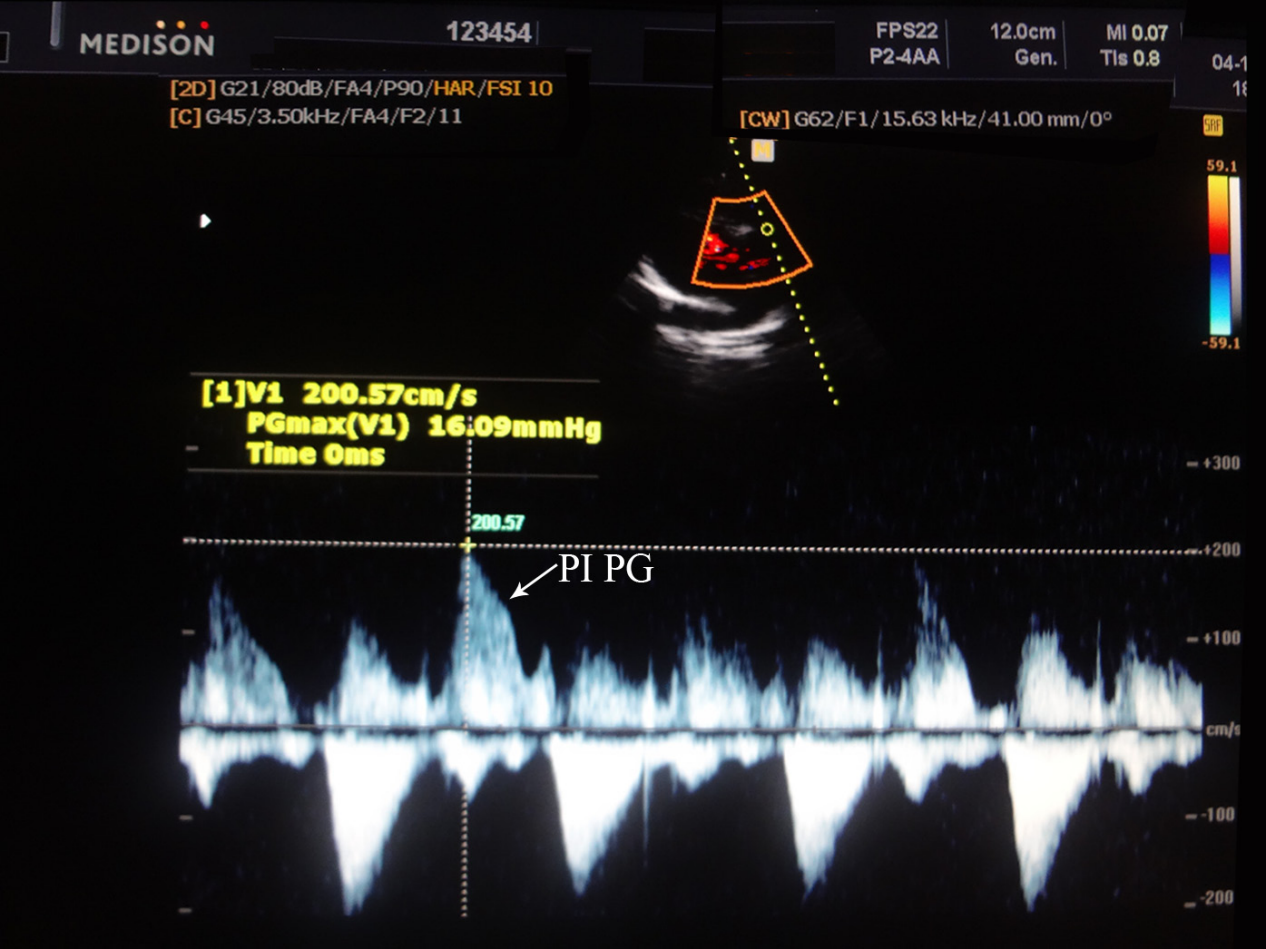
Continuous wave Doppler tracing of the pulmonary valve of the patient by transthoracic echocardiography at 2 years’ follow up shows mild pulmonary valve insufficiency with a 16 mmHg pressure gradient.
